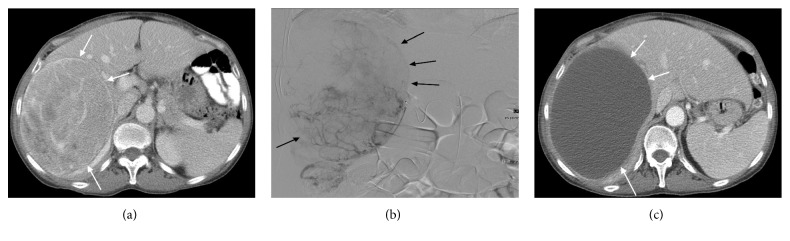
(a) CT scan of a patient with metastatic SFT in the liver prior to embolization demonstrating a 15.5 cm hypervascular mass in the right lobe of the liver (white arrows). (b) Fluoroscopic image of the same patient during hepatic artery embolization demonstrating a large hypervascular tumor in the right lobe of the liver (black arrows). (c) CT scan of the same patient one-month postembolization showing complete response as per mRECIST with no evidence of the residual viable tumor (white arrows).
